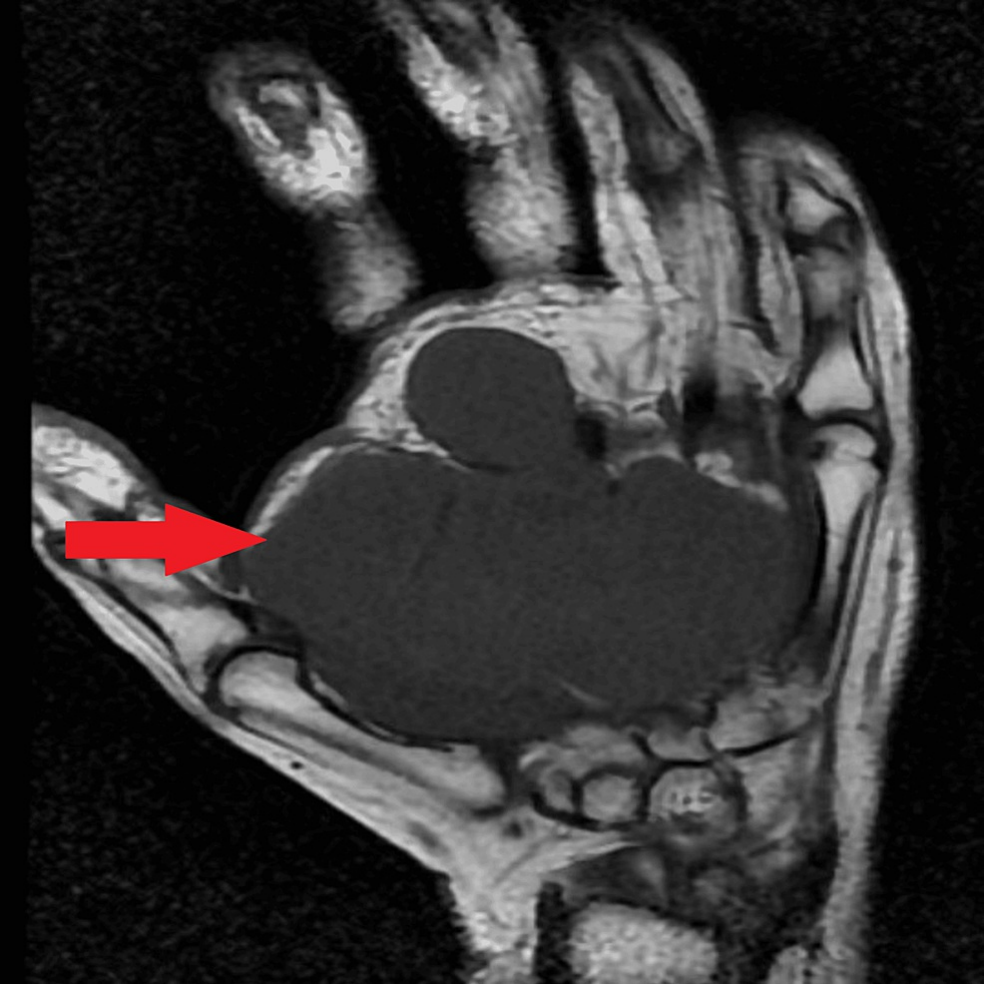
T1 weighted image of MRI MRI= Magnetic resonance imaging
